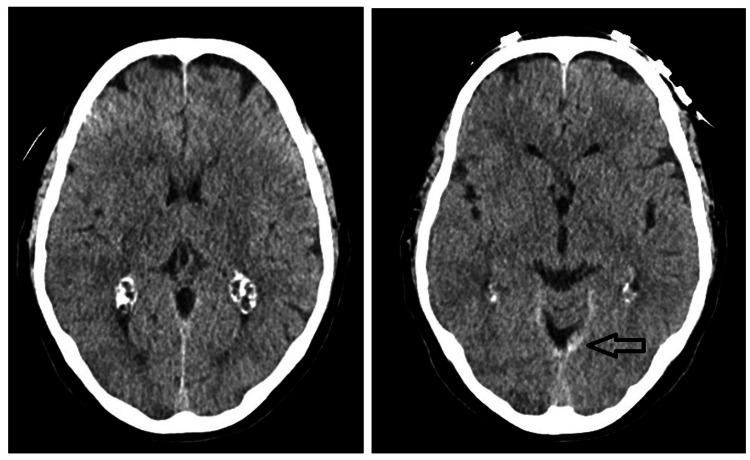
Post-operative brain computed tomography There is a slight decrease in the amplitude of the supratentorial ventricular system, associated with engorgement of the venous sinuses, interpeduncular hyperdensity, and hypodensity in the thalami (evoking a pseudohypoxic pattern). There is also the appearance of "pseudo-subarachnoid hemorrhage" (arrow).
